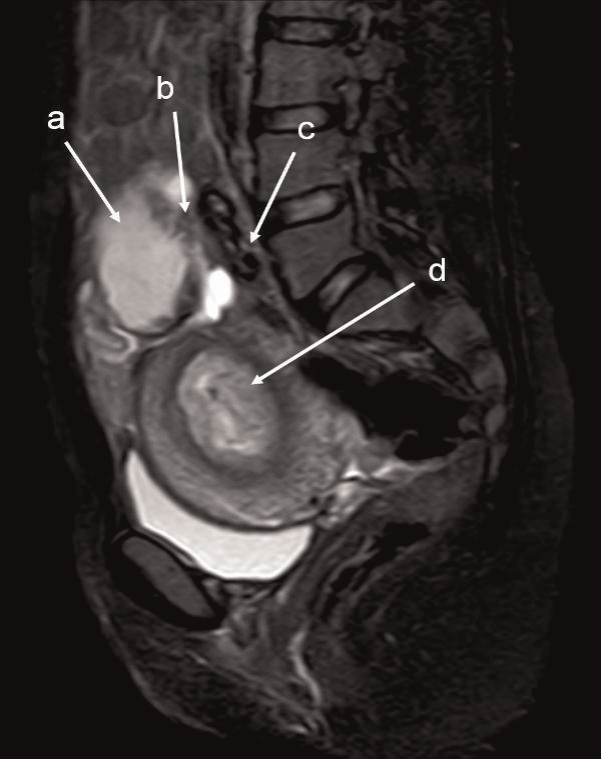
T2W SPAIR sagittal MRI of lower abdomen demonstrating the placental invasion. Placenta (a), invasion area (b), sigmoid colon (c), uterine cavity (d).
